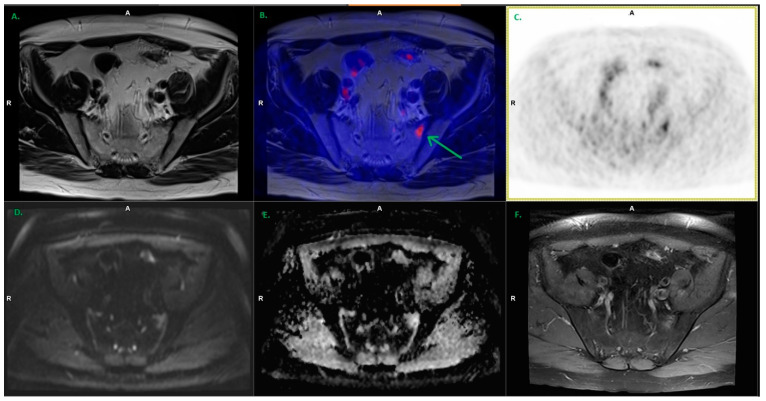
Moderate increased focal uptake of 18F-PSMA-1007 visible in the left ala of the sacrum (green arrow). Lesion considered benign based on appearance on MR images. In control PET/MR, performed in six months without any treatment, there was no evidence of increased PSMA uptake. ((A). T2_tse_tra, (B). Fused T2_tse_tra with PET AC, (C). PET AC, (D). DWI, (E). ADC map, (F). T1_vibe_CM—image after contrast agents’ injection).
